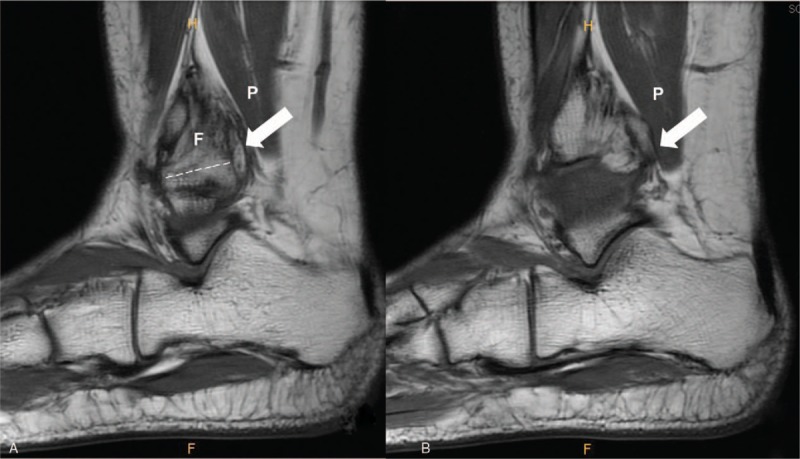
Preoperative magnetic resonance imaging shows the superior suture anchor (dotted line) (A) and mass-like lesion with a heterogeneous signal (white arrow) behind the site of anchor protrusion (B) on T1-weighted sagittal images (F, fibula, P, peroneus tendon).
